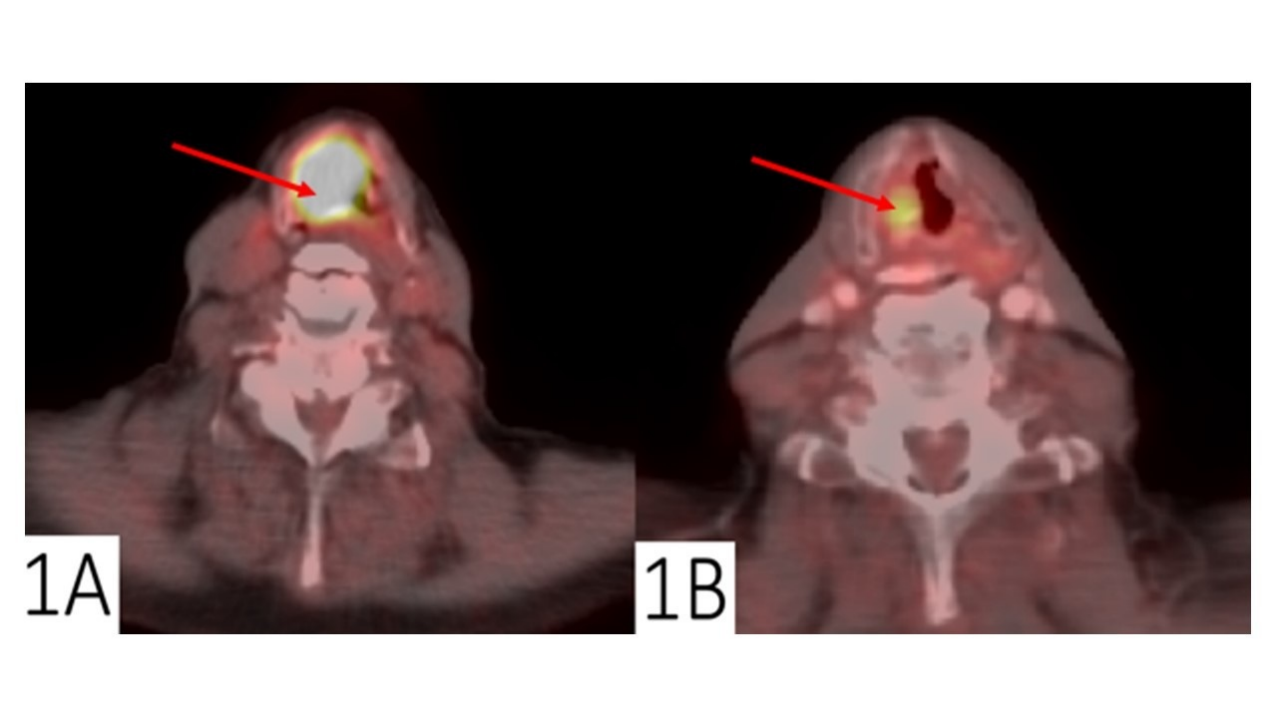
True positive post-treatment positron emission tomography/computed tomography (PET/CT) scan Pre-treatment and post-treatment positron emission tomography/computed tomography (PET/CT) for a laryngeal cancer. Representative matched axial PET/CT scan slices at approximately the level of the true glottis from a patient treated with definitive chemoradiation for initially staged T3N0M0, stage III, laryngeal cancer; (a) pre-treatment PET/CT scan with a CT mass measuring 2.7 x 2.3 cm at this level and SUVmax of 14 and; (b) post-treatment PET/CT scan with a residual CT mass measuring 1.4 x 1.1 cm and SUVmax of 5.9
